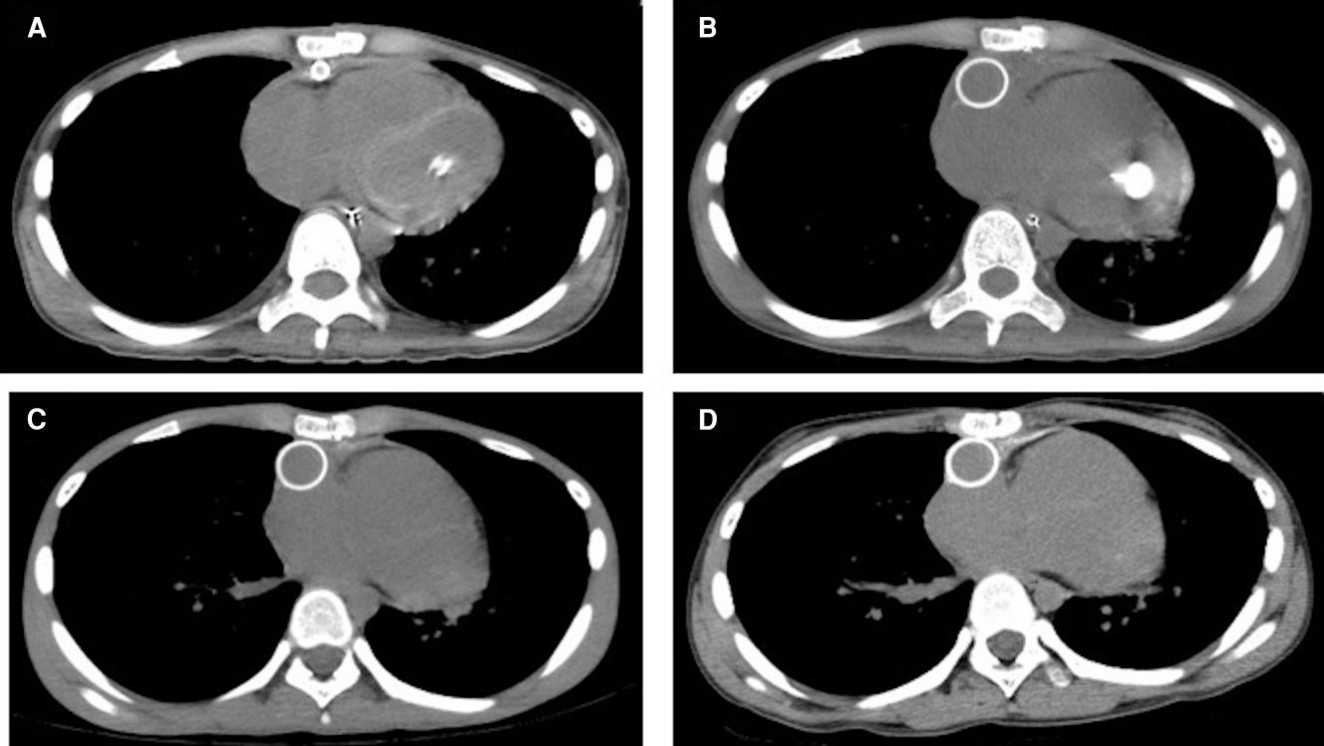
Case 1. A time course of myocardial calcification on CT at (*A*) 3 months, (*B*) 4 months, (*C*) 7 months, and (*D*) 14 months after admission. An annular dense shadow appeared on the LV wall 3 months after admission, but it regressed during follow-up.
